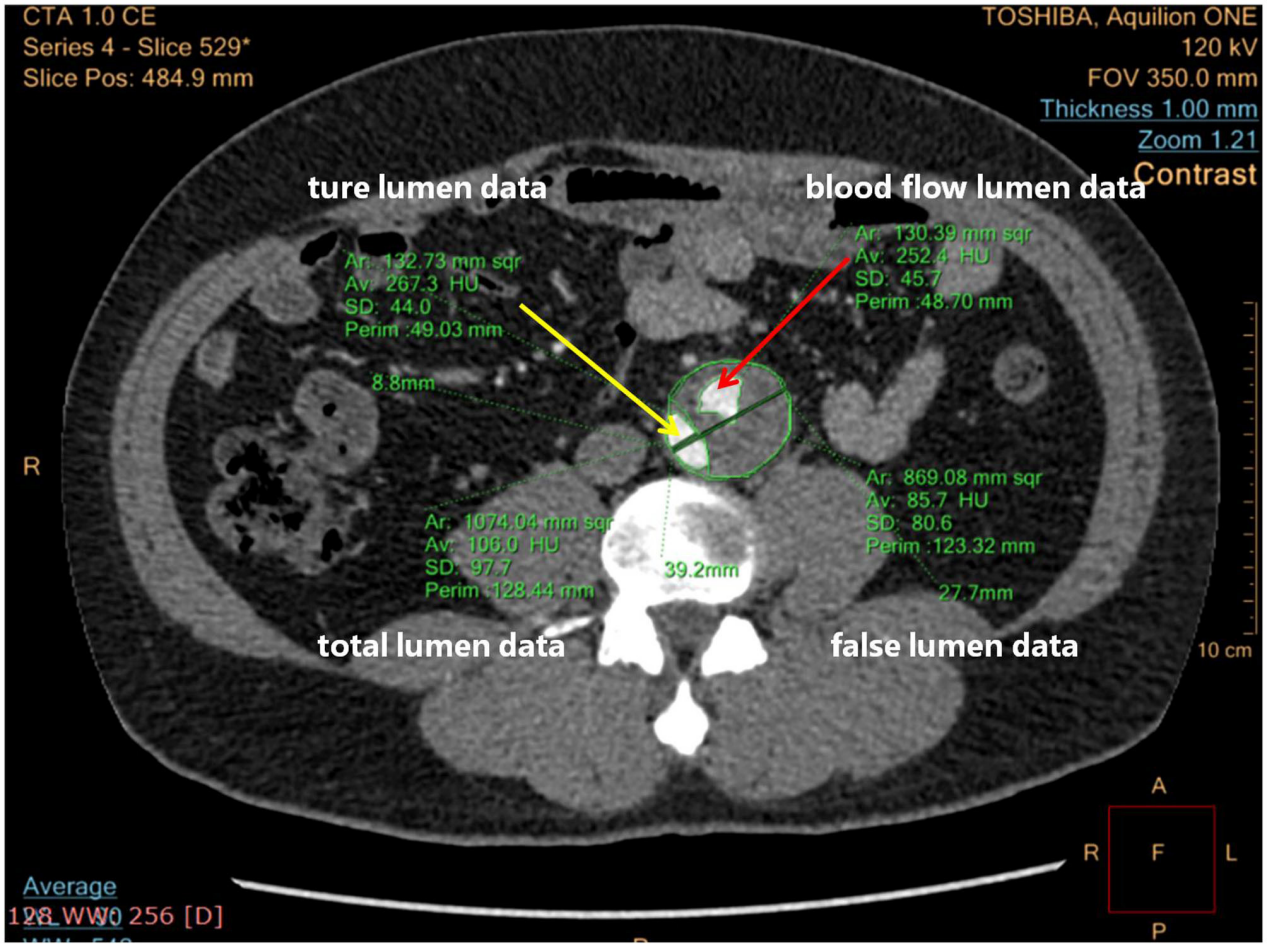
Computed tomographic angiography (CTA) cross-section measuring details blood flow lumen. The yellow arrow points to the true lumen; the red arrow points to the blood flow lumen.
